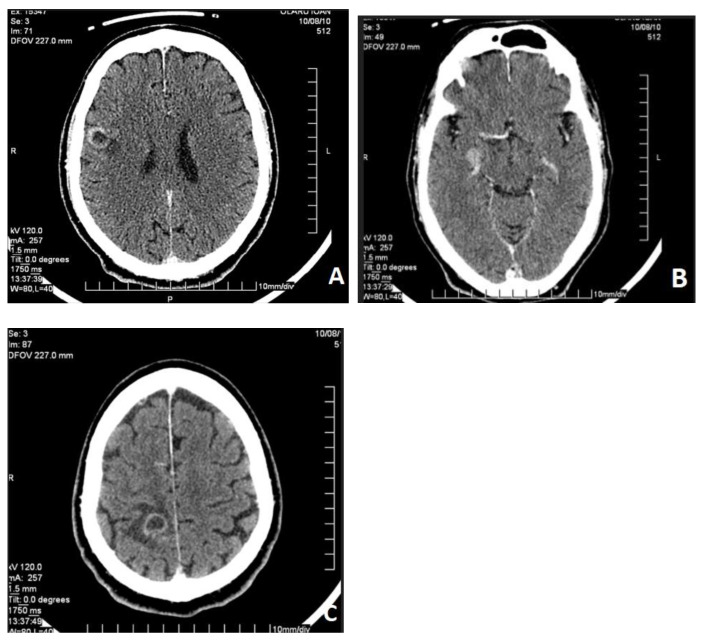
Axial postcontrast supratentorial cranial CT images show intraneural lesions with visible annular enhancement ((A,C), in the right frontal and parietal lobes) associated with perilesional edema, respectively, a homogeneously enhanced lesion ((B) in the right cerebellar peduncle).
